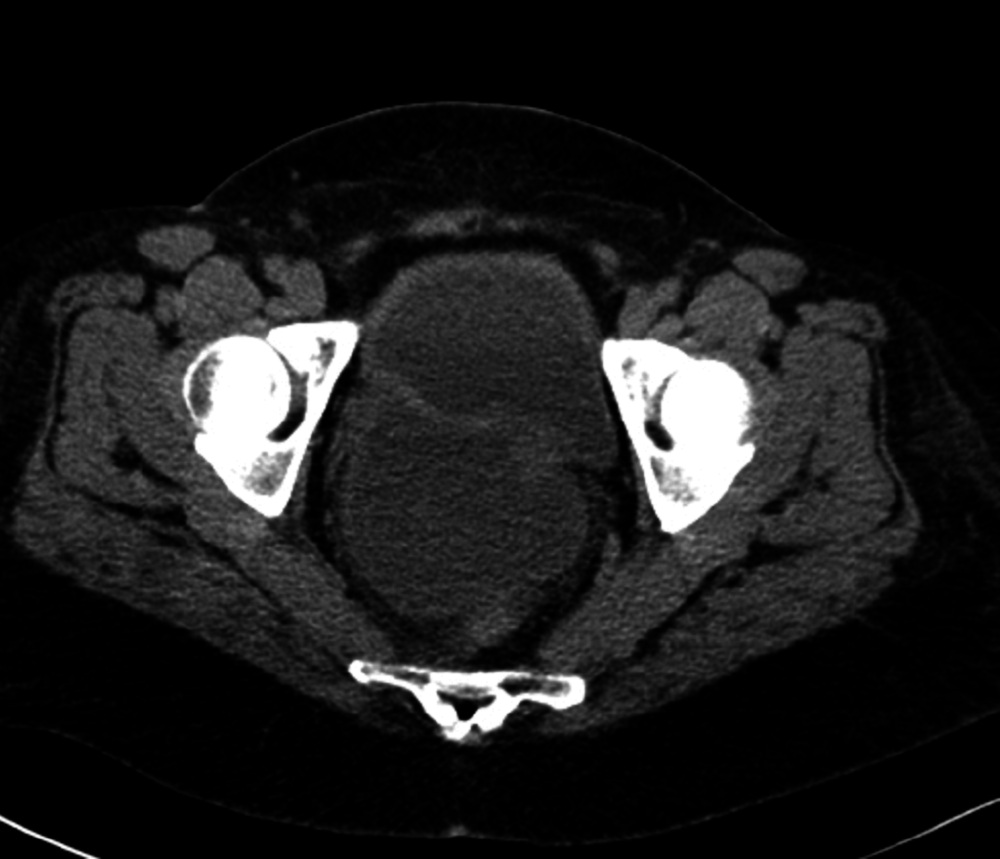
CT abdomen and pelvis without contrast showing bladder diverticulum, cannot rule out bladder rupture
